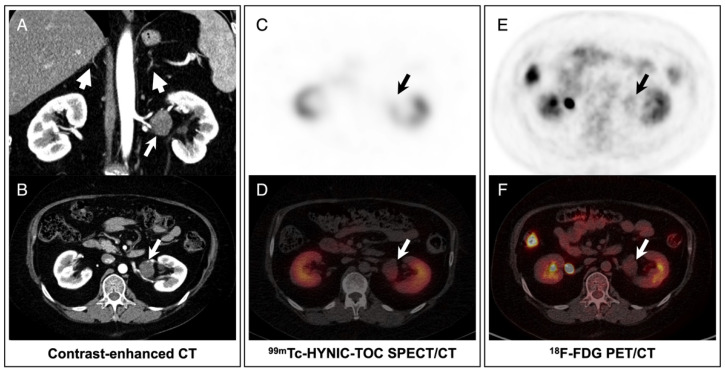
Imaging analysis of the patient. (A,B) Abdominal contrast-enhanced CT showed bilateral adrenal glands were atrophic without any nodules or hyperplasia (arrowheads), and a mass with mild enhancement in the left renal hilum (arrows). (C,D) ^99m^Tc-HYNIC-TOC SPECT/CT showed the mass was negative with somatostatin receptor imaging (arrows). (E,F) ^18^F-FDG PET/CT revealed mild FDG activity of the mass (arrows).
